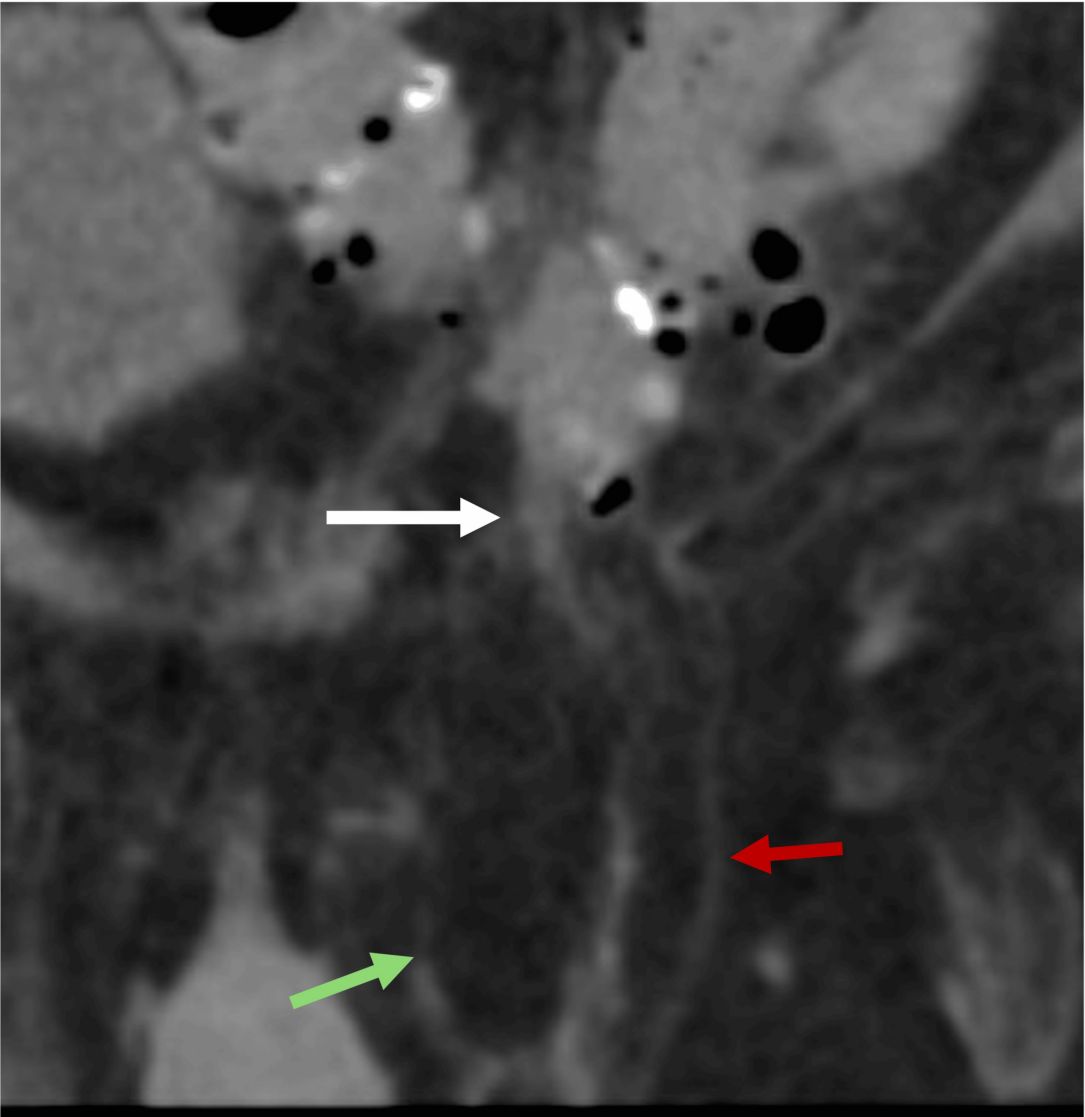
Coronal abdominal CT showing a pantaloon inguinal hernia Coronal abdominal CT showing a PIH with fat content. The direct hernia sac (green arrow) and indirect hernia sac (red arrow) are separated by the  inferior epigastric vessels (white arrow). The figure was created by the authors.
